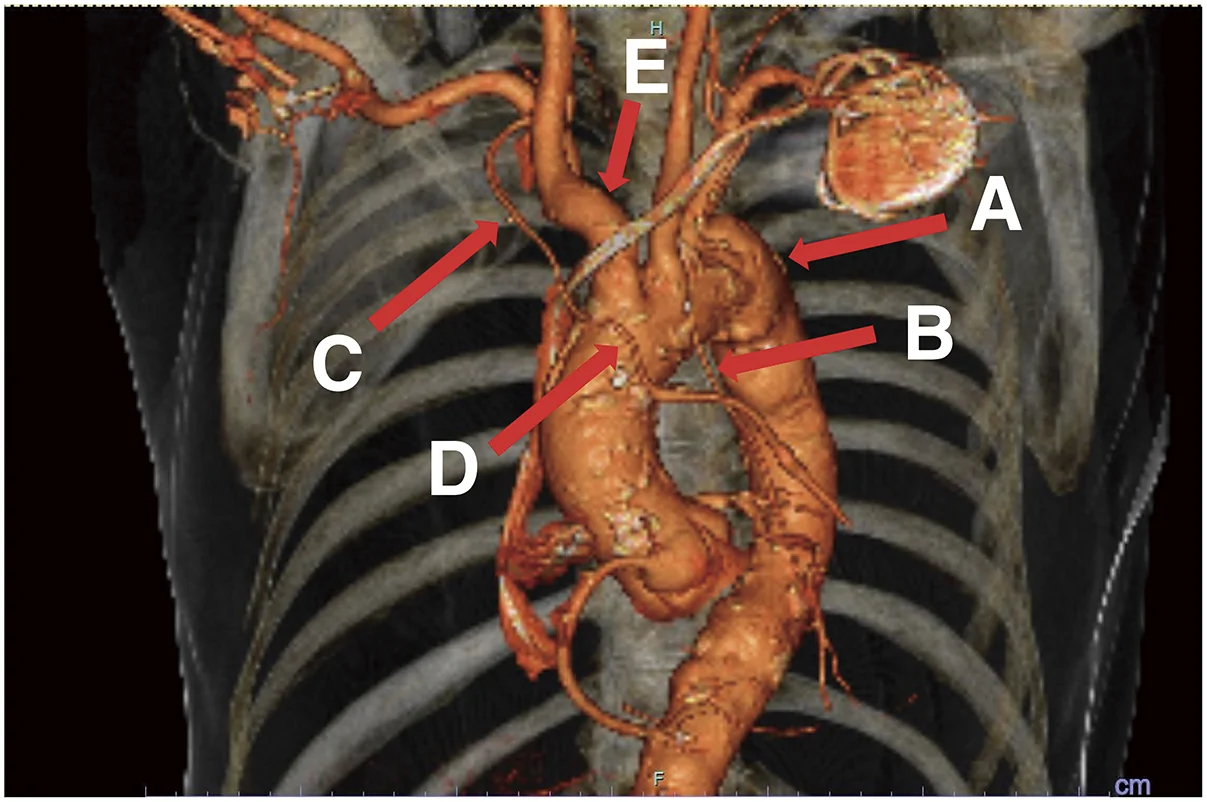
Preoperative reconstructive CT scan. (A) Aortic arch aneurysm. (B) Left internal thoracic artery (LITA). (C) Right internal thoracic artery (RITA). (D) Saphenous vein graft. (E) Brachiocephalic artery (BCA).
